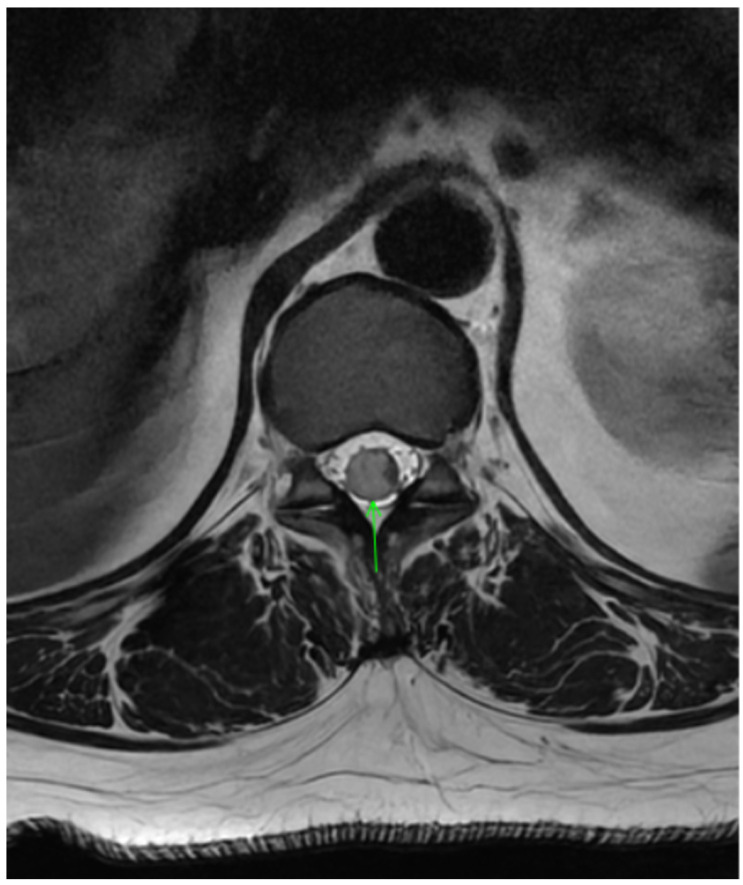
Thoracic MRI, T1 post-gadolinium obtained after PLEX demonstrated a decrease in lesion size, indicating partial radiologic response.
